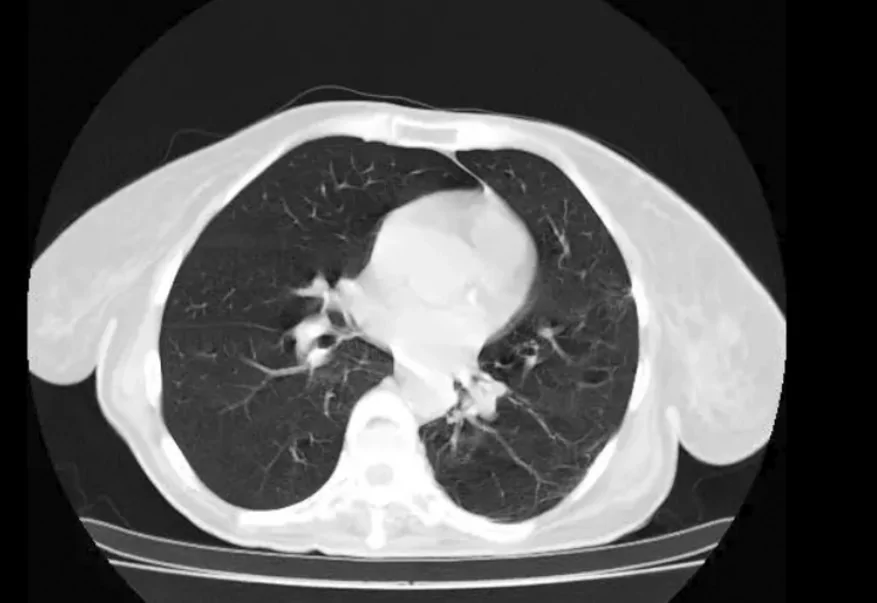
Chest CT scan. The chest CT image demonstrates clear lung fields with no evidence of consolidation, pleural effusion, atelectasis, or pulmonary embolism. The absence of significant pulmonary pathology supports a neuromuscular or central cause for the patient's hypoventilation.
